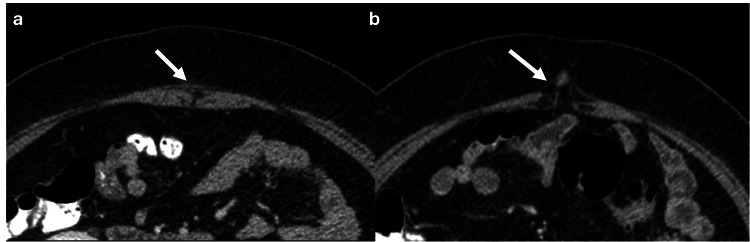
Axial abdominal CT scan with intravenous contrast showing post-abdominoplasty surgical changes (a) Axial CT scan shows the rectus abdominis muscles clumped along the midline (white arrow). (b) The umbilicus is remodeled, resembling a small tent (white arrow), these findings are consistent with post-abdominoplasty surgical changes. The figure was created by the authors.
